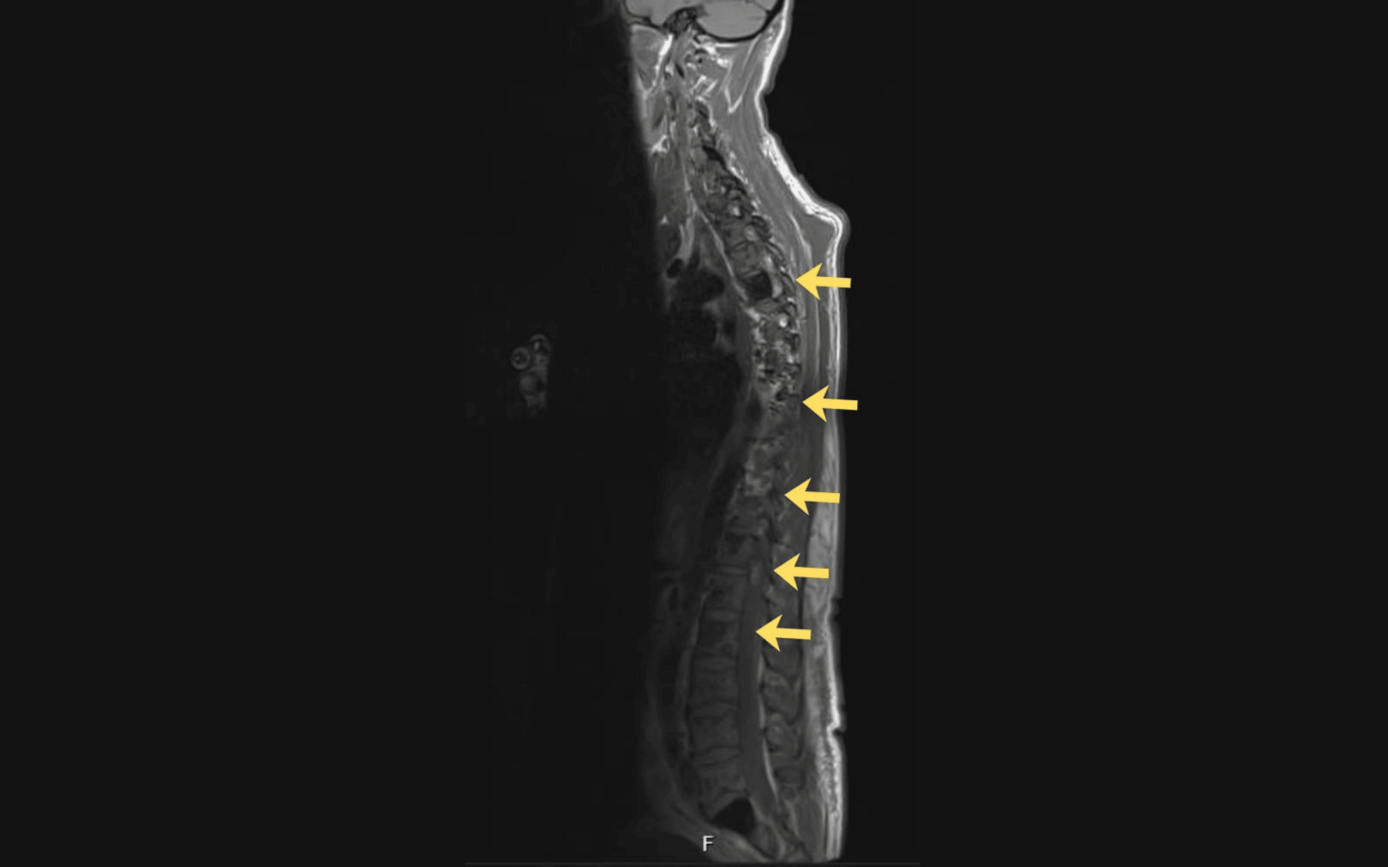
Whole-spine MRI (sagittal section) showing multiple sclerotic lesions in the vertebrae and visualised bones (arrows), with degenerative disc changes from C3 to C7 MRI: magnetic resonance imaging, C3-C7: the third through seventh cervical vertebrae.
